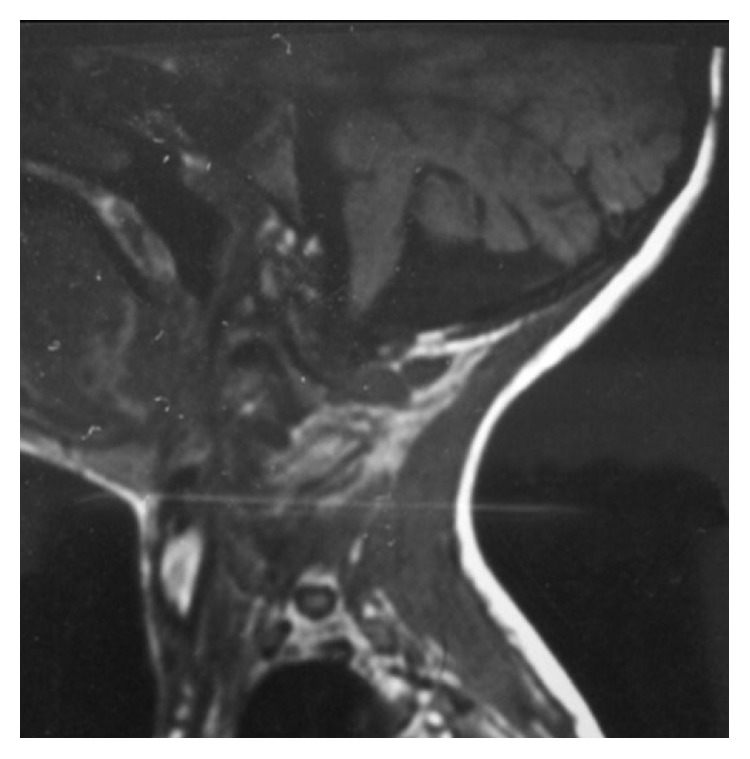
Preoperative MRI brainstem picture showing no abnormalities.
